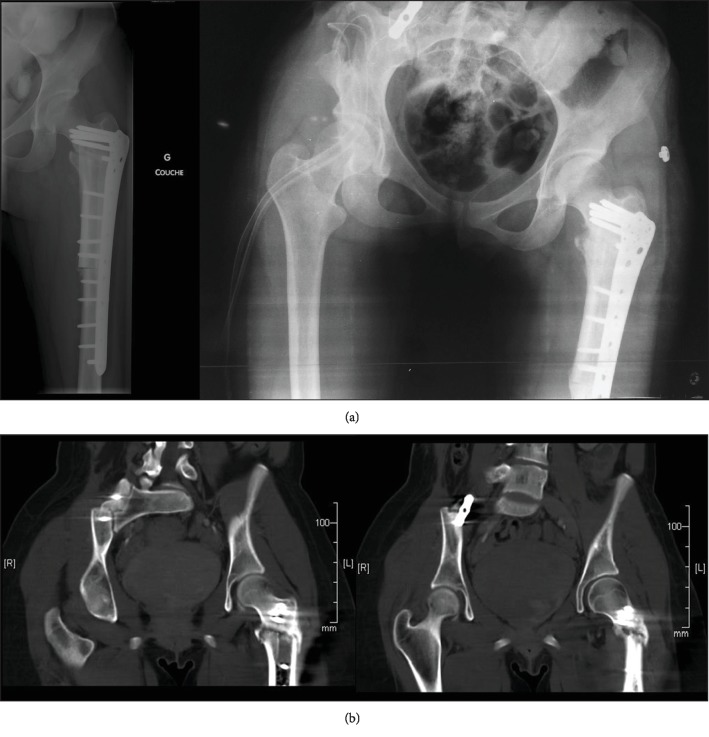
Postoperative imaging of the proximal left femur and of the right sacroiliac joint: (a) standard anteroposterior radiographs and (b) coronal CT scan.
